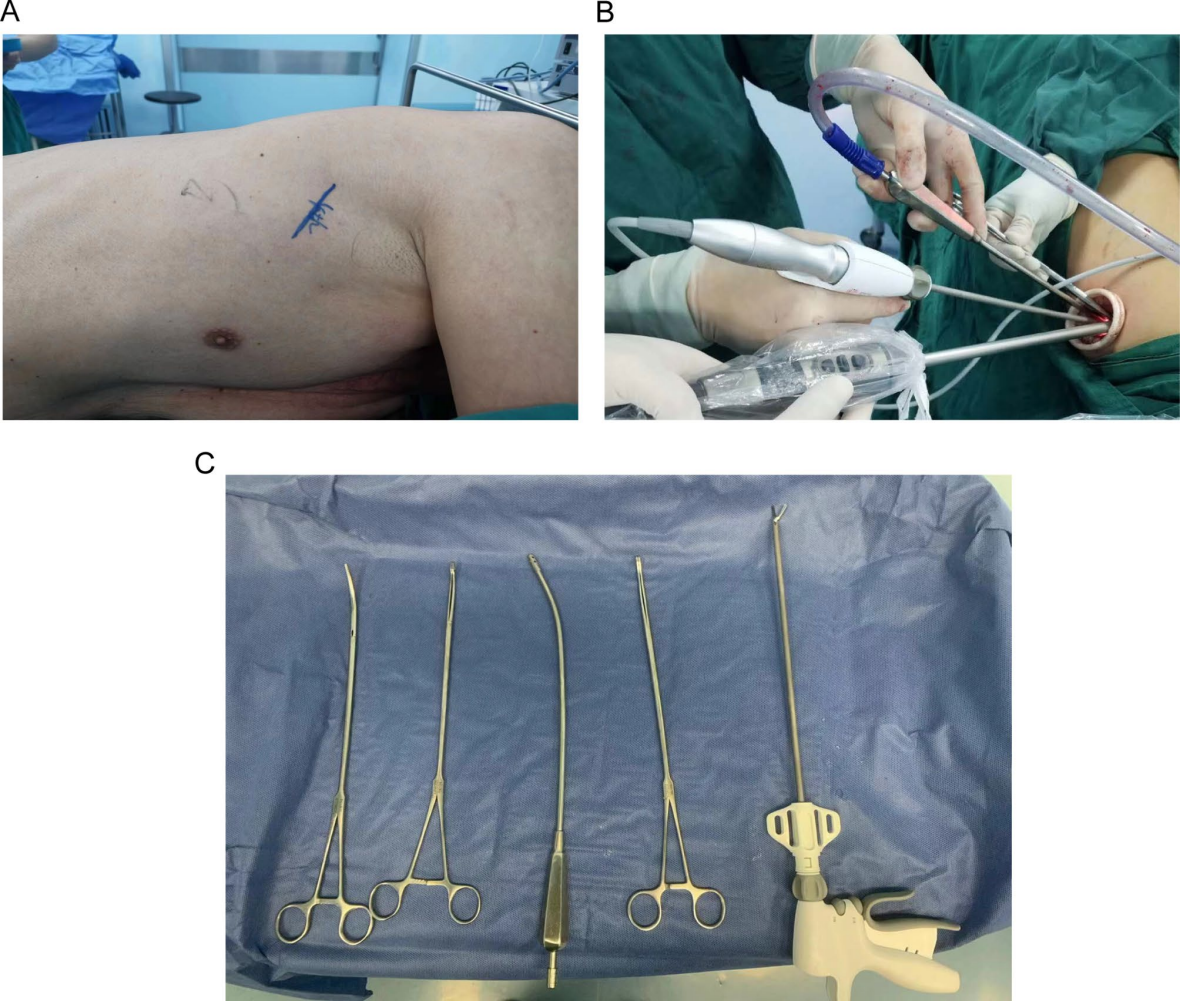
Single-port thoracoscopic minimally invasive esophagectomy in patients with esophageal squamous cell carcinoma. (A) The left lateral prone position was adopted, and the surgical incision was selected as the fourth intercostal of the posterior axillary line, with an incision length of 3 cm. (B) Position of instruments and operators in thoracic phase. (C) Special instruments used during the operation. From left to right: double-joint vascular forceps for separating tissue; double-joint snakehead forceps exposing surgical field or transporting gauze; elbow suction apparatus for sucking blood and smoke; double-joint ring forceps for immobilizing soft tissues or exposing surgical field; ultrasonic harmonic scalpel for separating tissue or stopping bleeding.
